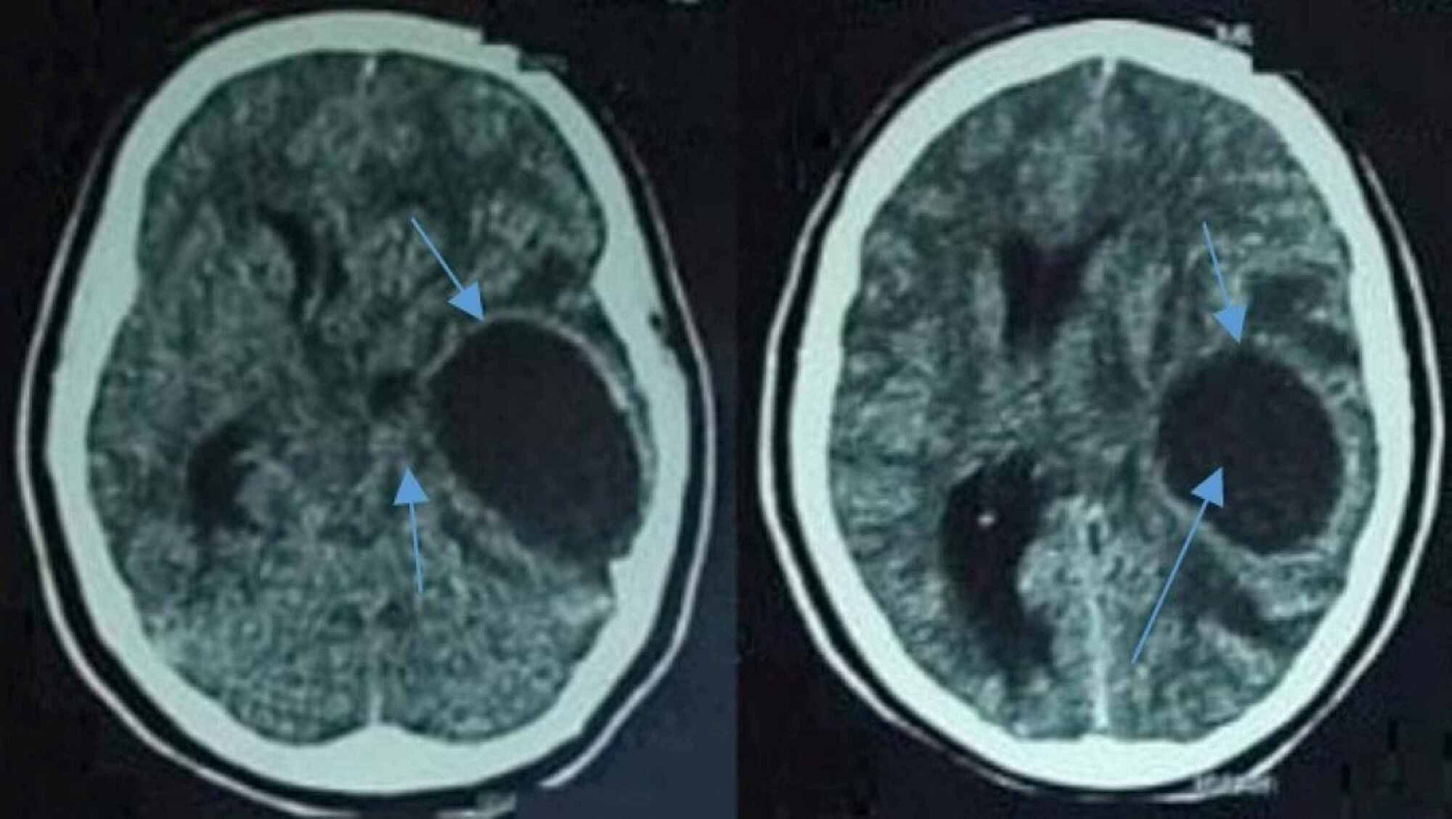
Axial images of the CT brain showing an enhancing cystic lesion with perilesional edema and midline shift (blue arrows). CT: Computed tomography
